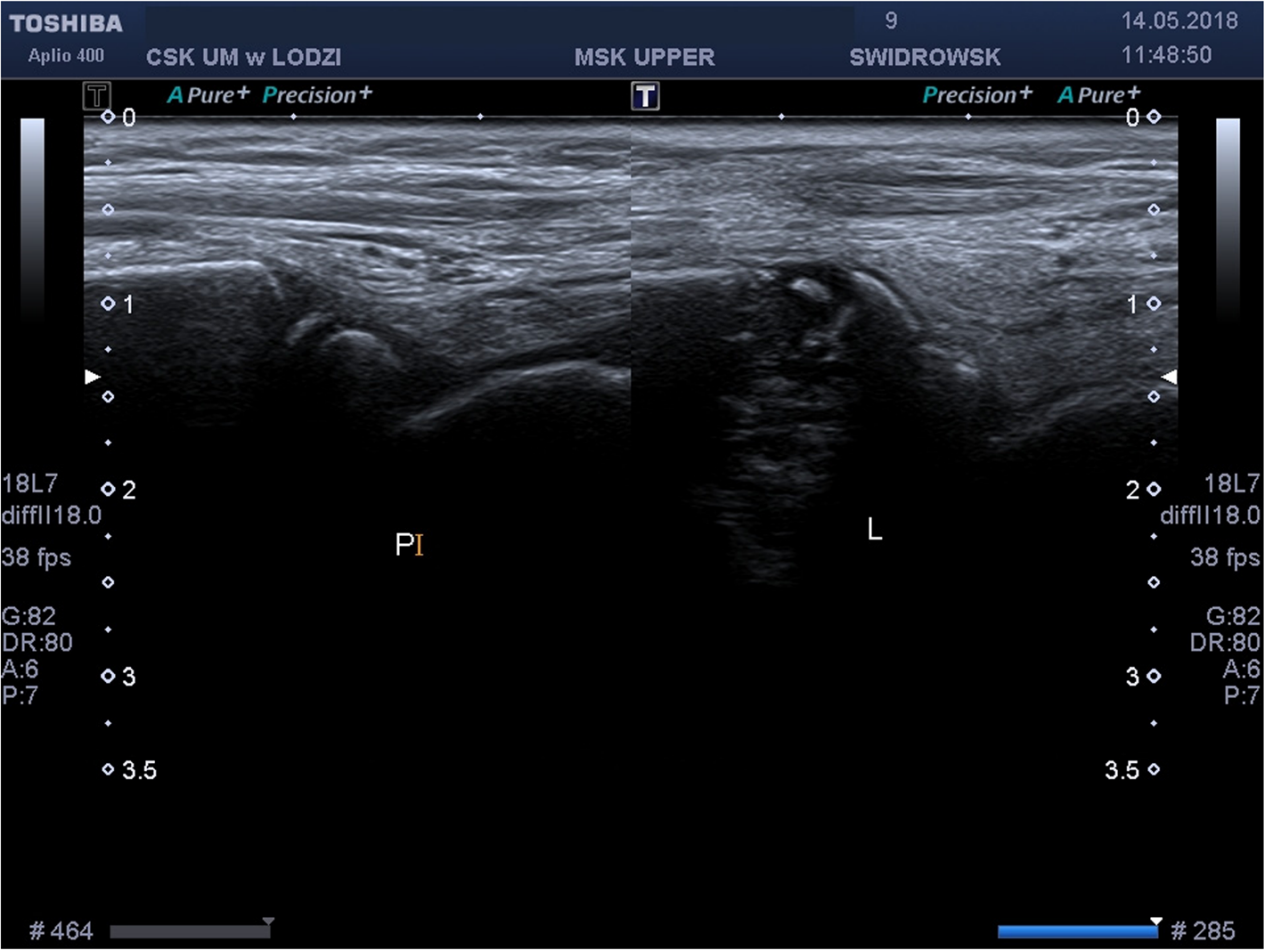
Ultrasound examination of an ankle area showing destruction of distal metaphysis of the left Tibia
